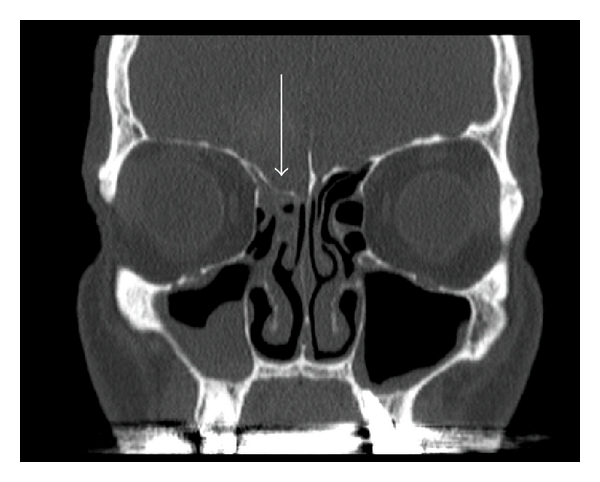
Ethmoid roof is lower on the right side (arrow). Coronary CT scan of a patient with chronic sinusitis.
